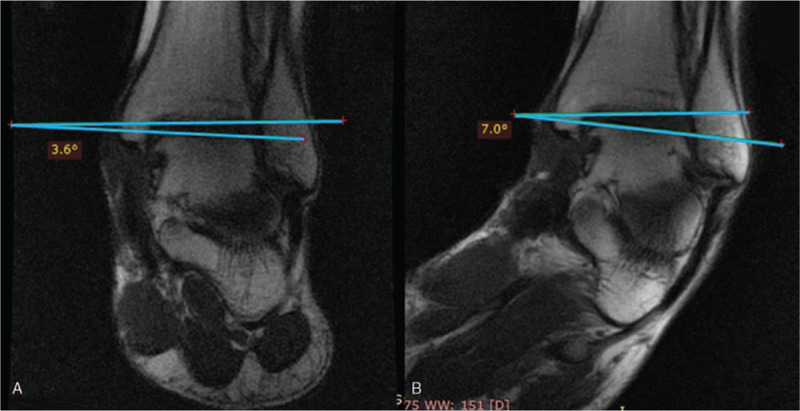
PATD assessment for varus stress test under MRI control. (A) Rest position and (B) after inversion stress (the blue lines show the angle between the distal surface of the tibia and the proximal surface of the talar dome).
